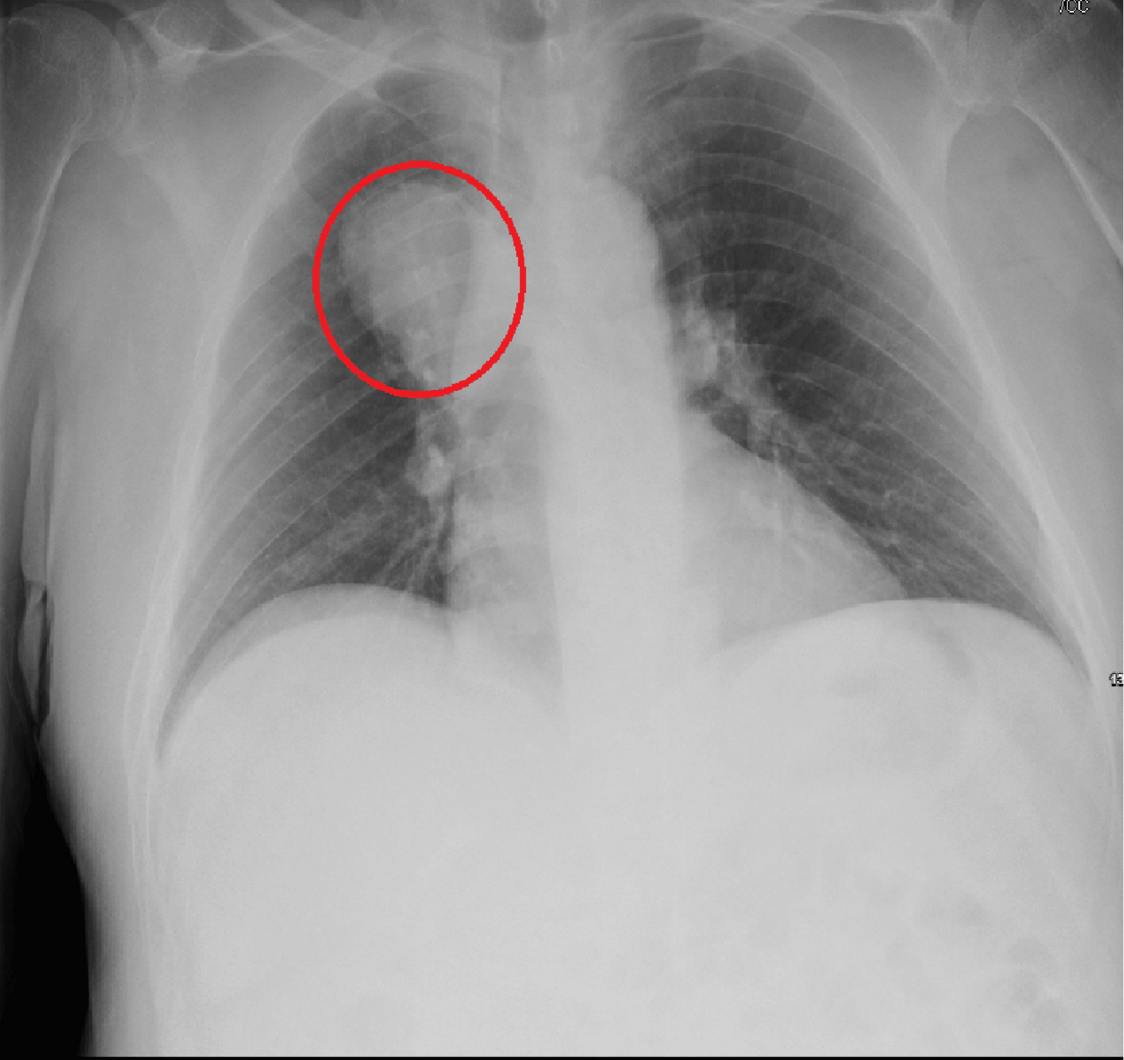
Initial chest X-ray There is a large indeterminate approximately 8-cm right perihilar/suprahilar mass indicated by the red circle; otherwise, the heart appears to be of normal size, and the lungs are clear, with no pneumothorax or significant pleural effusion noted.
